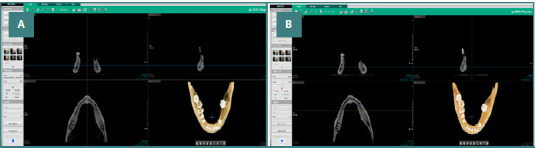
Effect of exposure settings on absorbed dose and image quality in CBCT imaging. A, Image obtained using the default exposure of the device (kVp: 94, mA: 8.1), resulting in an absorbed dose of 654.47 µGy with an image quality (IQ) level of 'very good'. B, Image obtained using the manually selected exposure setting (kVp: 80, mA: 4, absorbed dose: 198.5 µGy), which resulted in the lowest absorbed dose among all scan protocols while maintaining a very good IQ level.
